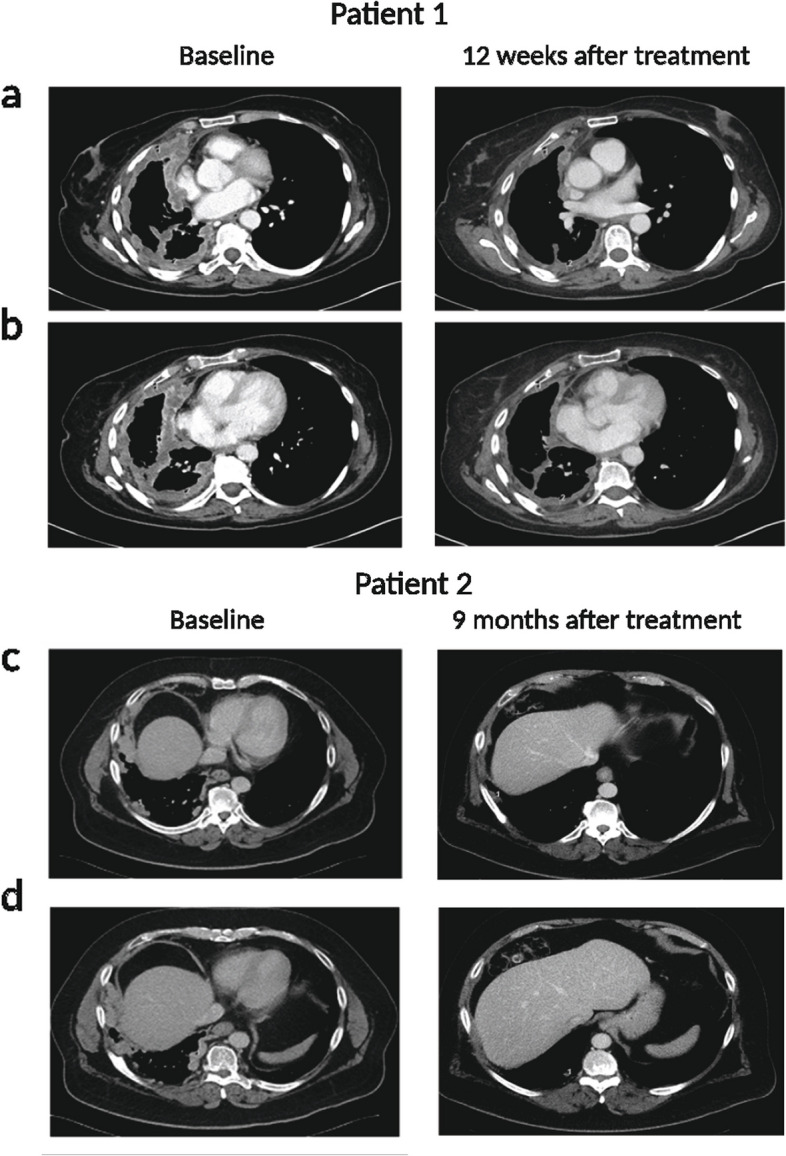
Radiological responses to tislelizumab and ociperlimab for the two PM patients. 1A and 1B represent PET-CT images of patient number one, and 1C and 1D correspond to patient number two. A Axial section at the level of the right superior pulmonary vein at baseline (1 = 18 mm, 2 = 10 mm) and 12 weeks after treatment (1 = 5 mm, 2 = 5 mm) (B) Axial section at T9 vertebra level at baseline (1 = 10 mm, 2 = 11 mm) and 12 weeks after treatment (1 = 8 mm, 2 = 8 mm). C Axial section at the level of right 9.^th^ rib at baseline (1 = 14 mm) and 9 months after treatment (1 = 8 mm). D Axial section at the level of T9 vertebra at baseline (1 = 14 mm) and 9 months after treatment (1 = 6 mm)
